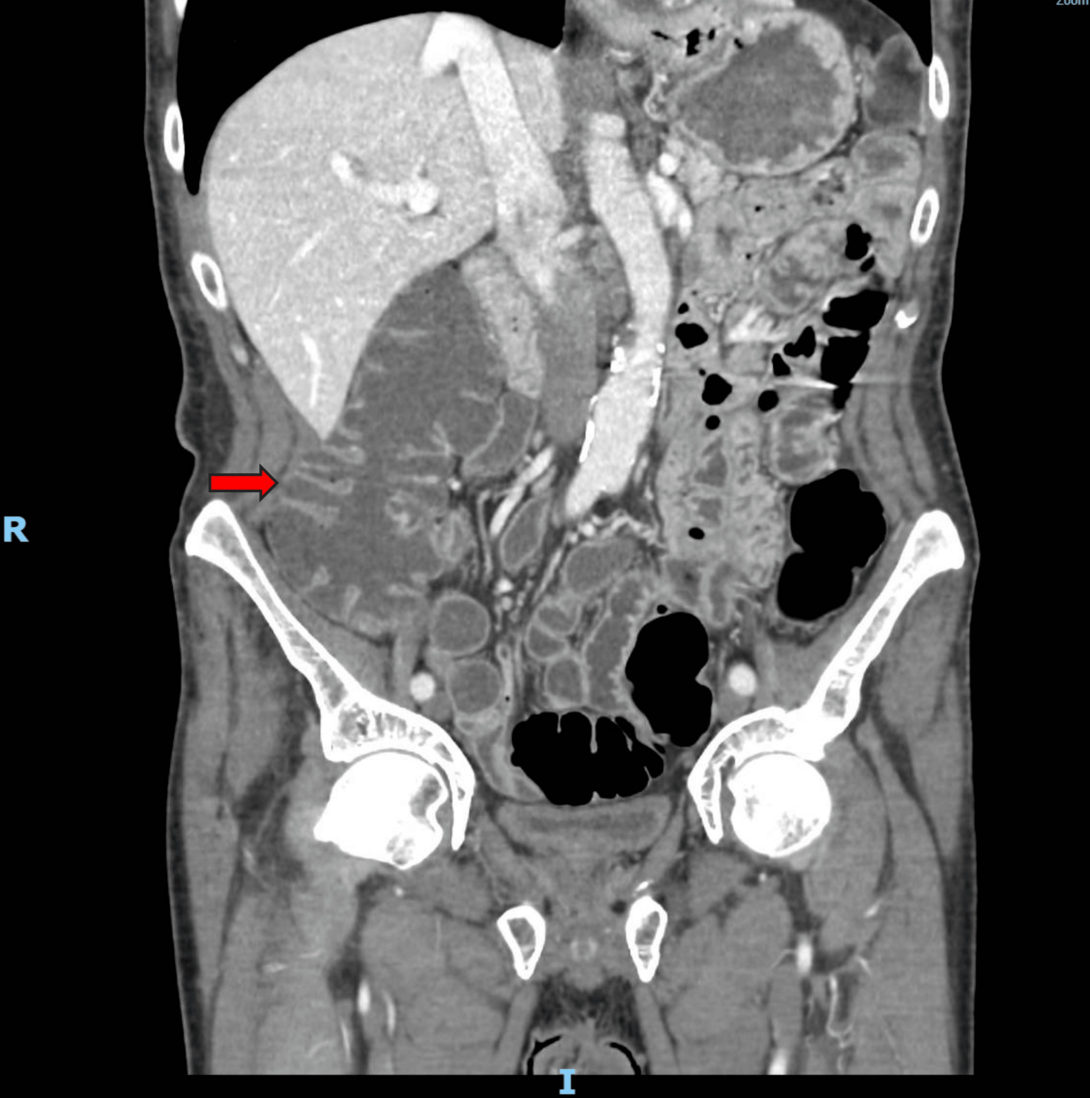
CT of the abdomen and pelvis with intravenous contrast In this CT, there is evidence of fluid in the ascending colon (red arrow).
